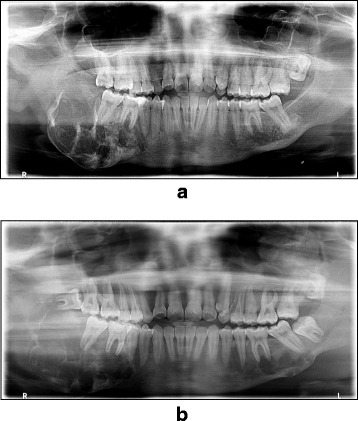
Panoramic x-ray of the patient’s teeth showing right mandibular swelling and an impacted left lower wisdom tooth. a Preoperative x-ray. b Postoperative intralesional steroid injection and removal of the left lower wisdom tooth
